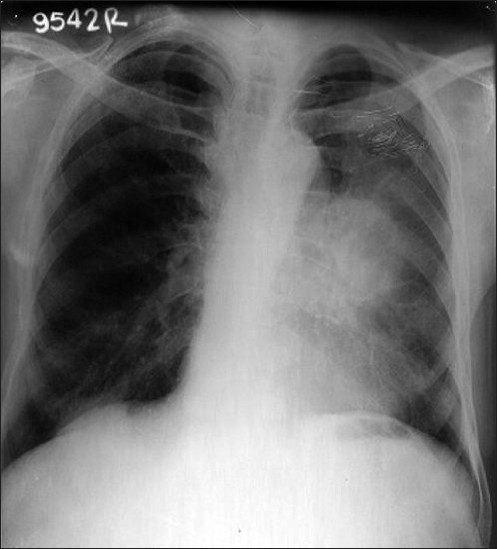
X-ray chest showing mass in left lung mid zone
